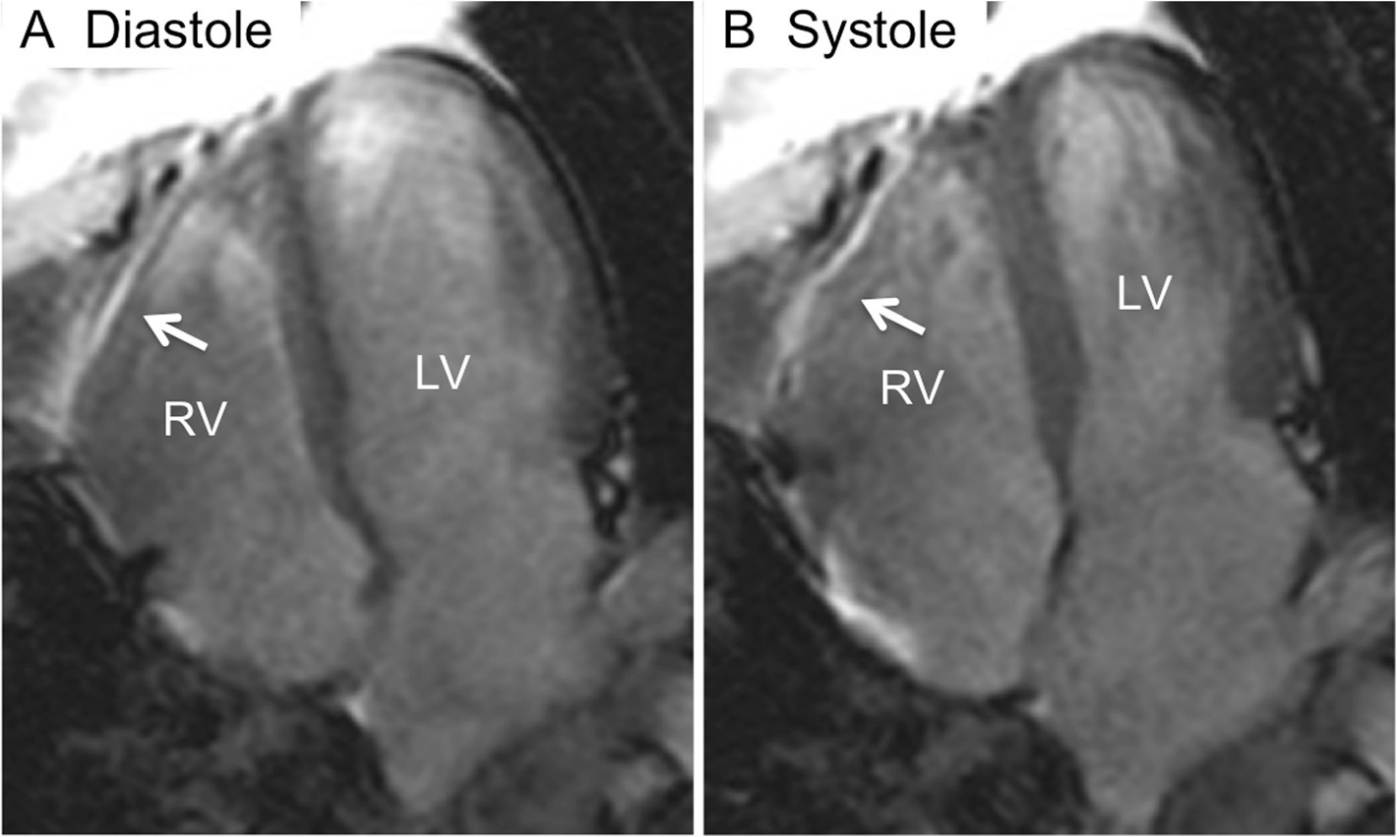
Functional (cine) cardiac magnetic resonance images in the four-chamber image plane in diastole (A) and systole (B). The images show poor contraction of the right ventricle (RV) free wall (arrows in A and B), but normal contraction of the left ventricle (LV).
